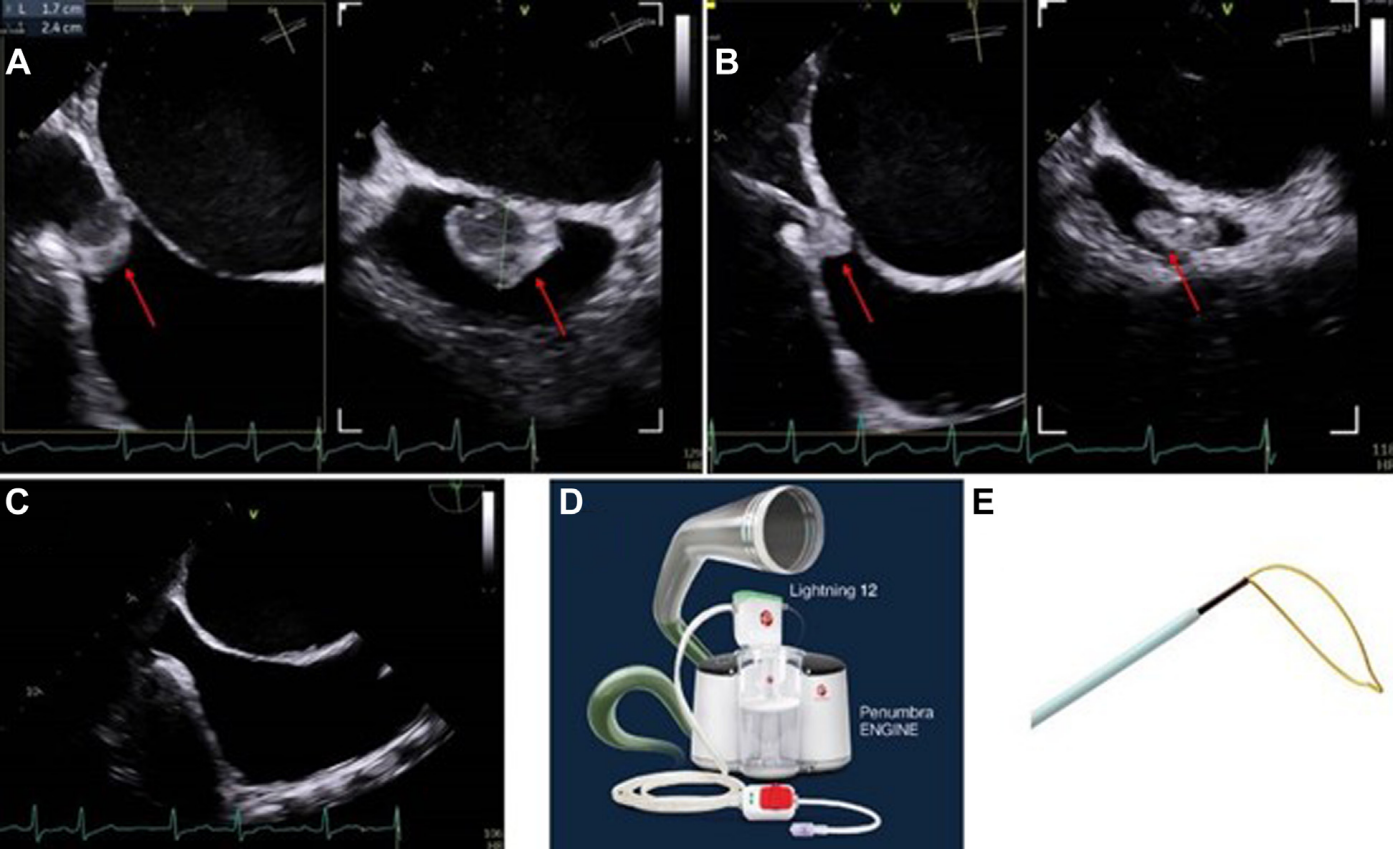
The QATAR Procedure (A) Transesophageal echocardiographic (TEE) bicaval view of a moderate-sized, pedunculated right atrial mass (red arrow) adherent to the interatrial septum. (B) TEE images of the Lightning Flash Indigo system, engaging the mass with negative pressure aspiration. (C) TEE images of right atrium after tumor extraction. (D) Lightning Flash Indigo system. (E) A loop snare device (Medtronic).
